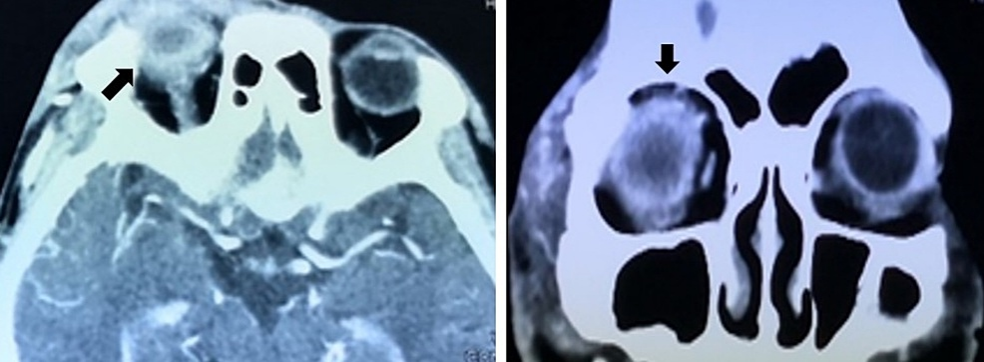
The axial and coronal views of the computed tomography scan revealed ill-defined hyperdense lesions within the right globe (arrow), with irregular septation
